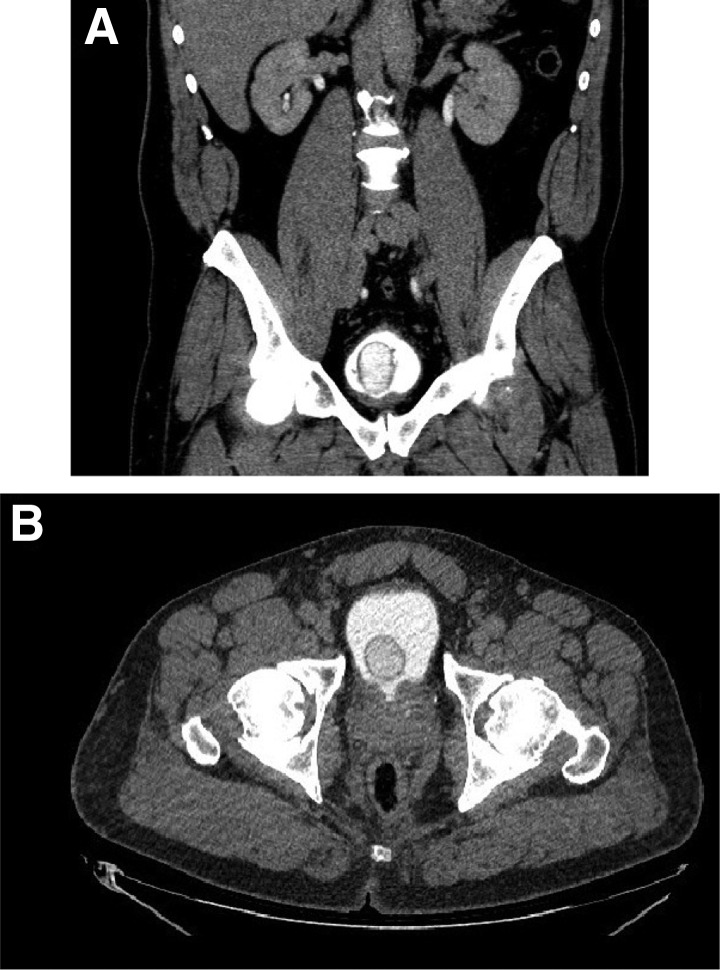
Computed tomography scan with intravenous contrast of the abdomen and pelvis; delayed imaging. Coronal cross-sectional imaging shows the right ureterocele within the bladder (A). Transverse imaging demonstrates the proximity of the ureterocele to the bladder neck and outlet (B).
